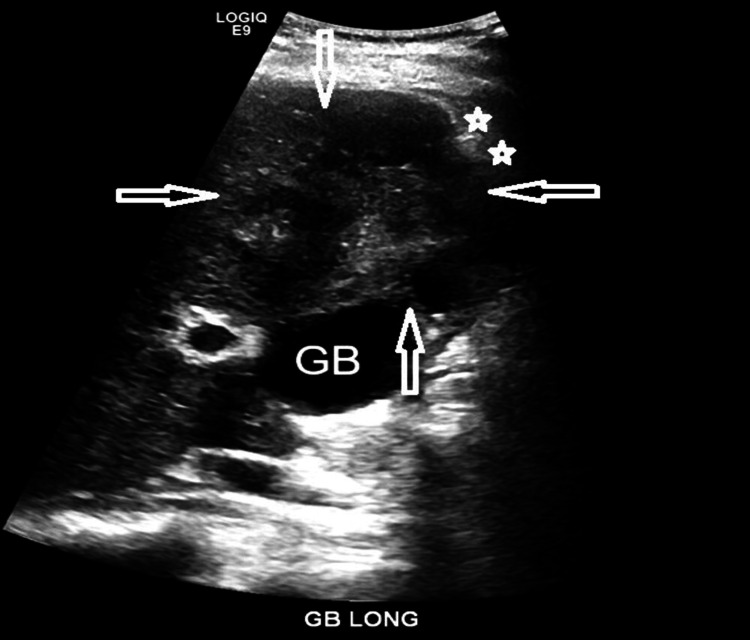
Ultrasonography image of the liver and gallbladder (GB) demonstrates a heterogeneous lesion (arrows) on segment IV/V as heterogeneous area with liver capsular retraction (stars)
